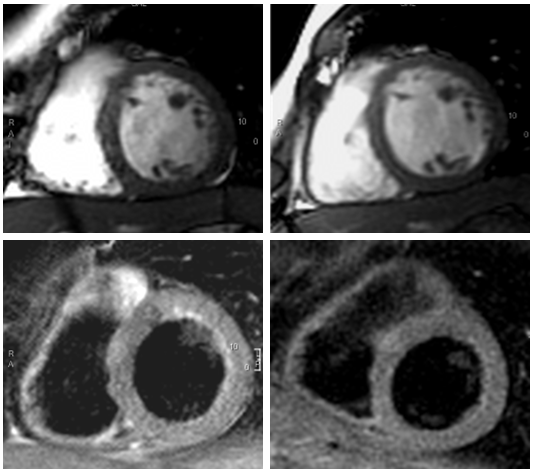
CMR images in the acute (left) and convalescent (right) phase of myocarditis. Upper left: SSFP-cine images showing increased LV mass compared to follow-up (upper right). Lower left: T2-weighted STIR images with higher signal intensity of myocardium compared to follow-up (lower right). [SSFP: steady-state free precession].
